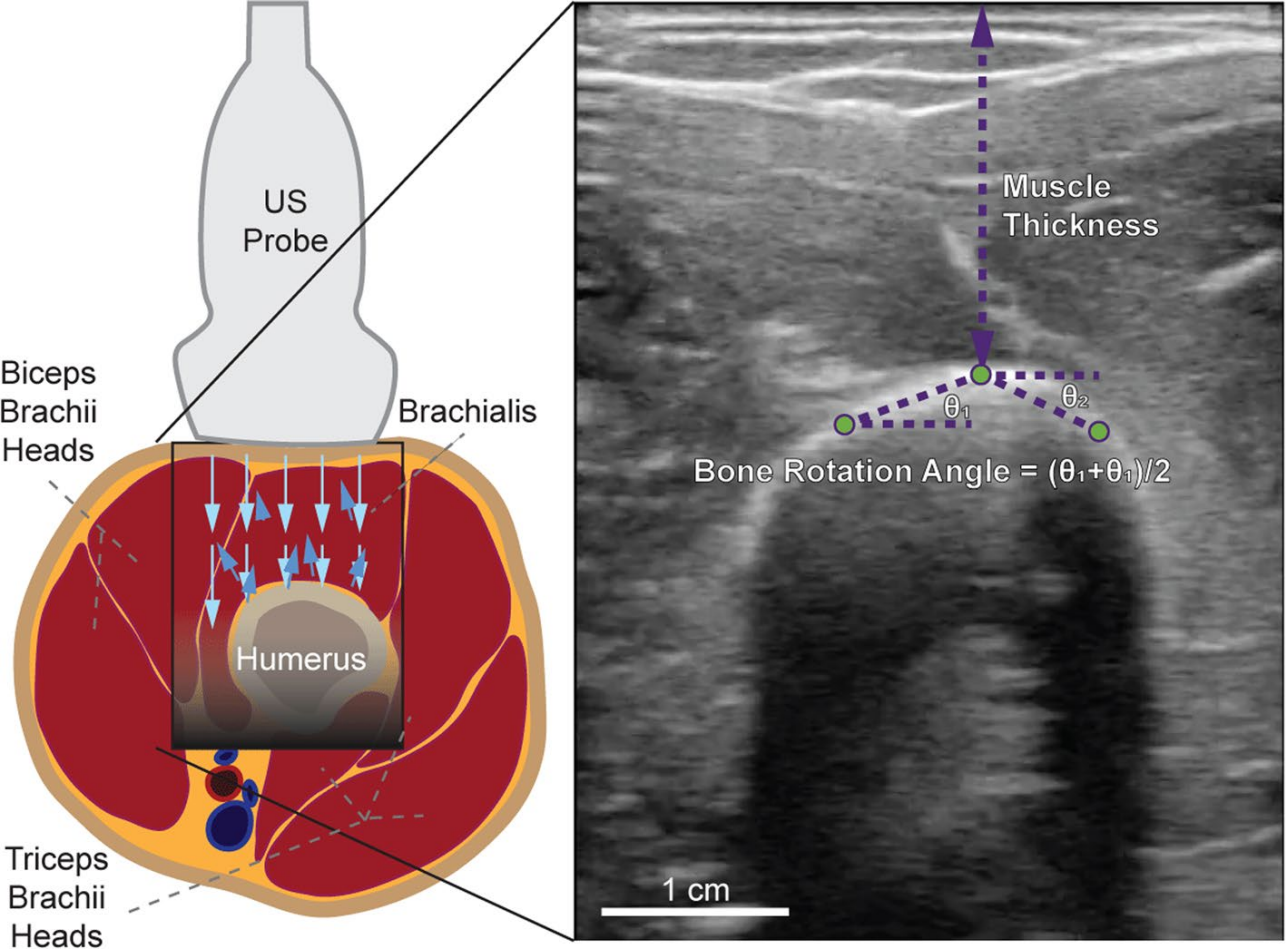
Ultrasound imaging setup. Ultrasound probe position on the upper arm for imaging the brachialis and the long head of the biceps brachii (left). A representative US image (right) showing how features such as muscle thickness and bone rotation can be estimated from tracking specific points of interest in ultrasound videos
